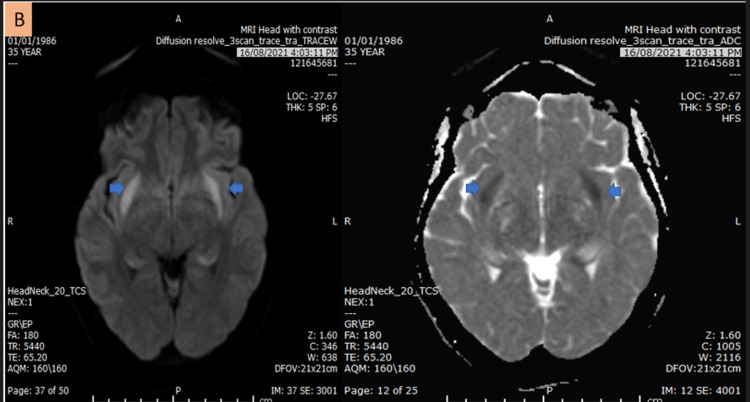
Head MRI axial diffusion and ADC map demonstrate symmetrical claustrum diffusion restriction (blue arrow). MRI: magnetic resonance imaging; ADC: apparent diffusion coefficient
